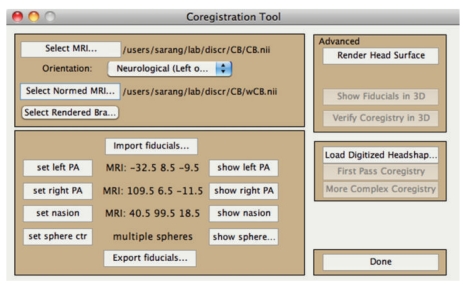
Coregistration Toolbox GUI allows inclusion of subject's MRI, fiducials to be loaded or set manually, spatially normalized MRI or rendered surface (created via SPM8), surface mesh, and digitized headshape points.
